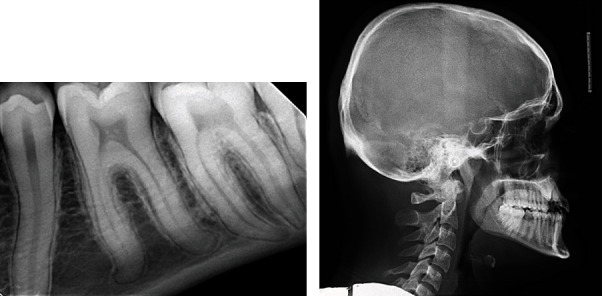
Periapical radiograph shows long roots of teeth #35, 36, 37 (a) and lateral cephalometric radiograph of the patient (b).
